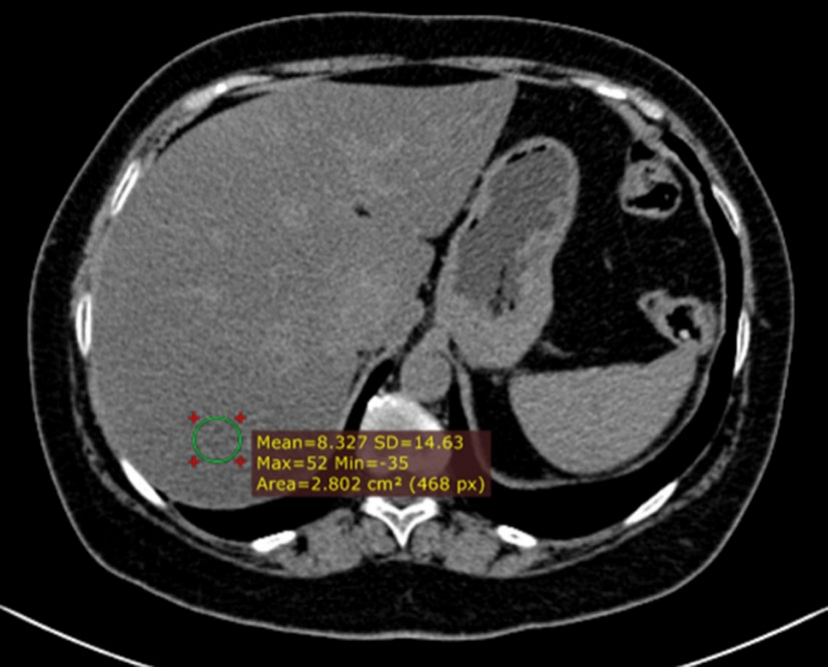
59-year-old woman with history of hysterectomy, bilateral salpingo-oophorectomy and segmental resection of distal left ureter for uterine leiomyosarcoma, and tumor relapse at first CT follow-up after 3 months from surgery and then treated with chemotherapy (doxurubicine-dacarbazine). Precontrast CT at 3 months after chemotherapy shows low attenuation of the liver parenchyma with an absolute value of 8 HU, consistent with post-ChT steatosis
